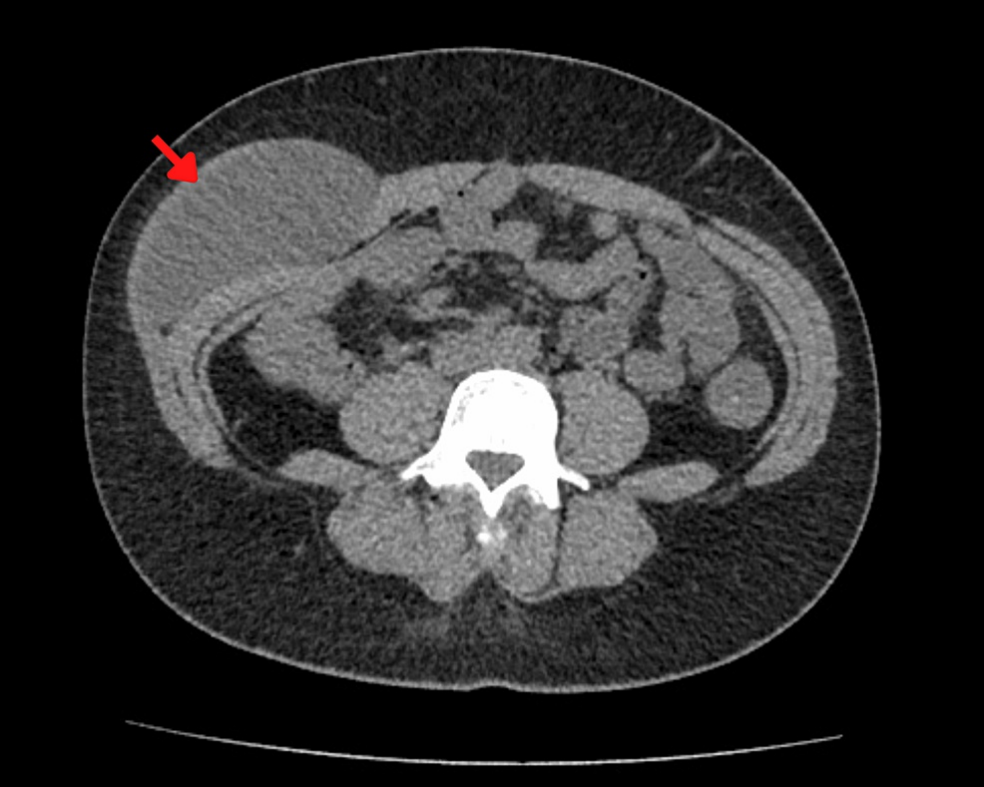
An axial view of abdominal computed tomography shows a mass between the external and internal oblique muscles on the right side (red arrow)
